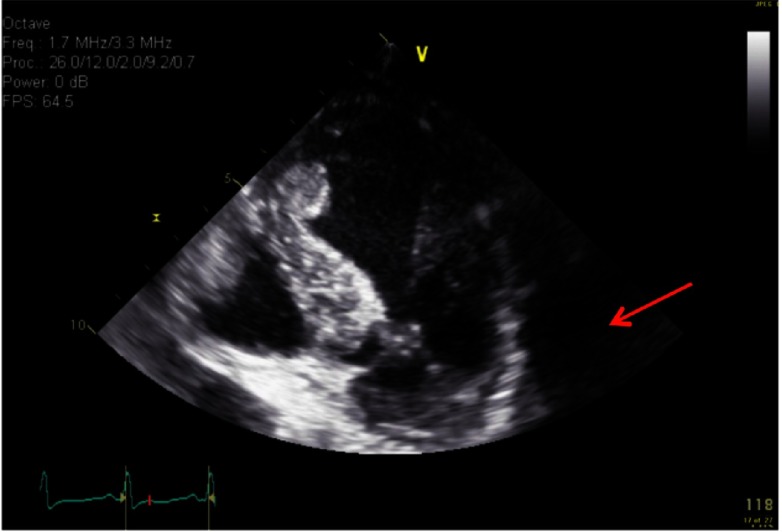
Echocardiography showing SAM of mitral valve with LVOT obstruction.
